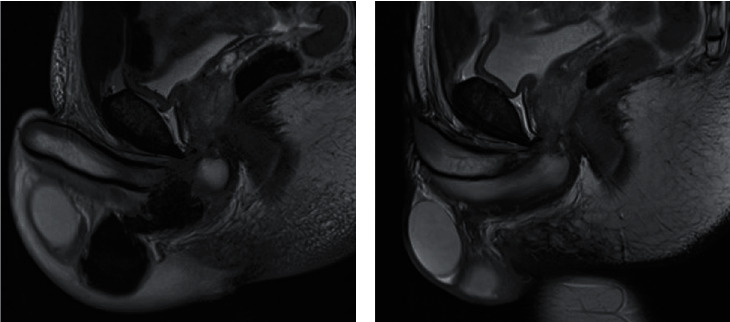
T2-weighted sagittal pelvis MRI; (a) large hematoma spreading in the scrotum; (b) complete resolution at six weeks.
